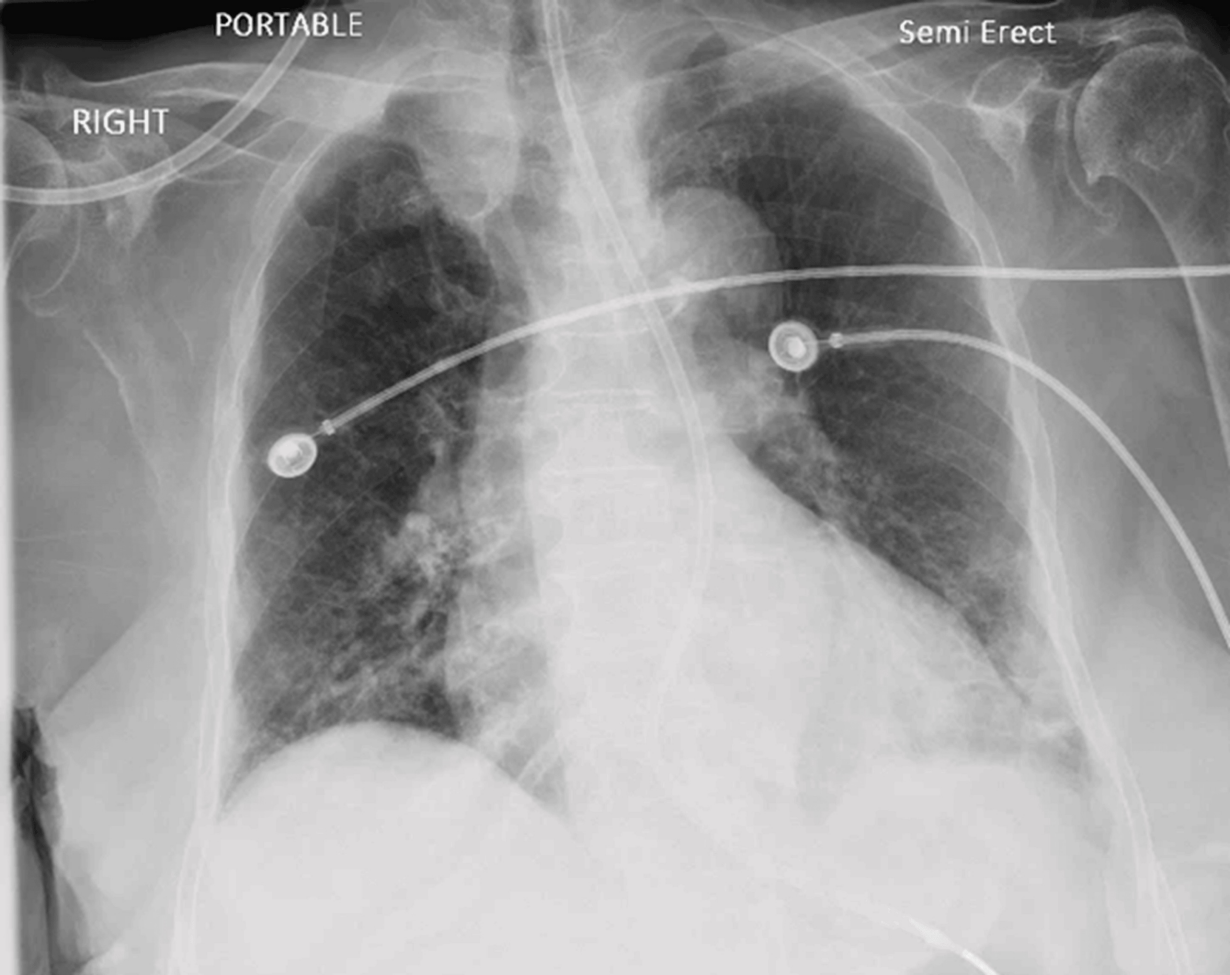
X-ray of the chest prior to discharge Chest x-ray performed before hospital discharge showed improvement in pulmonary infiltrates.
